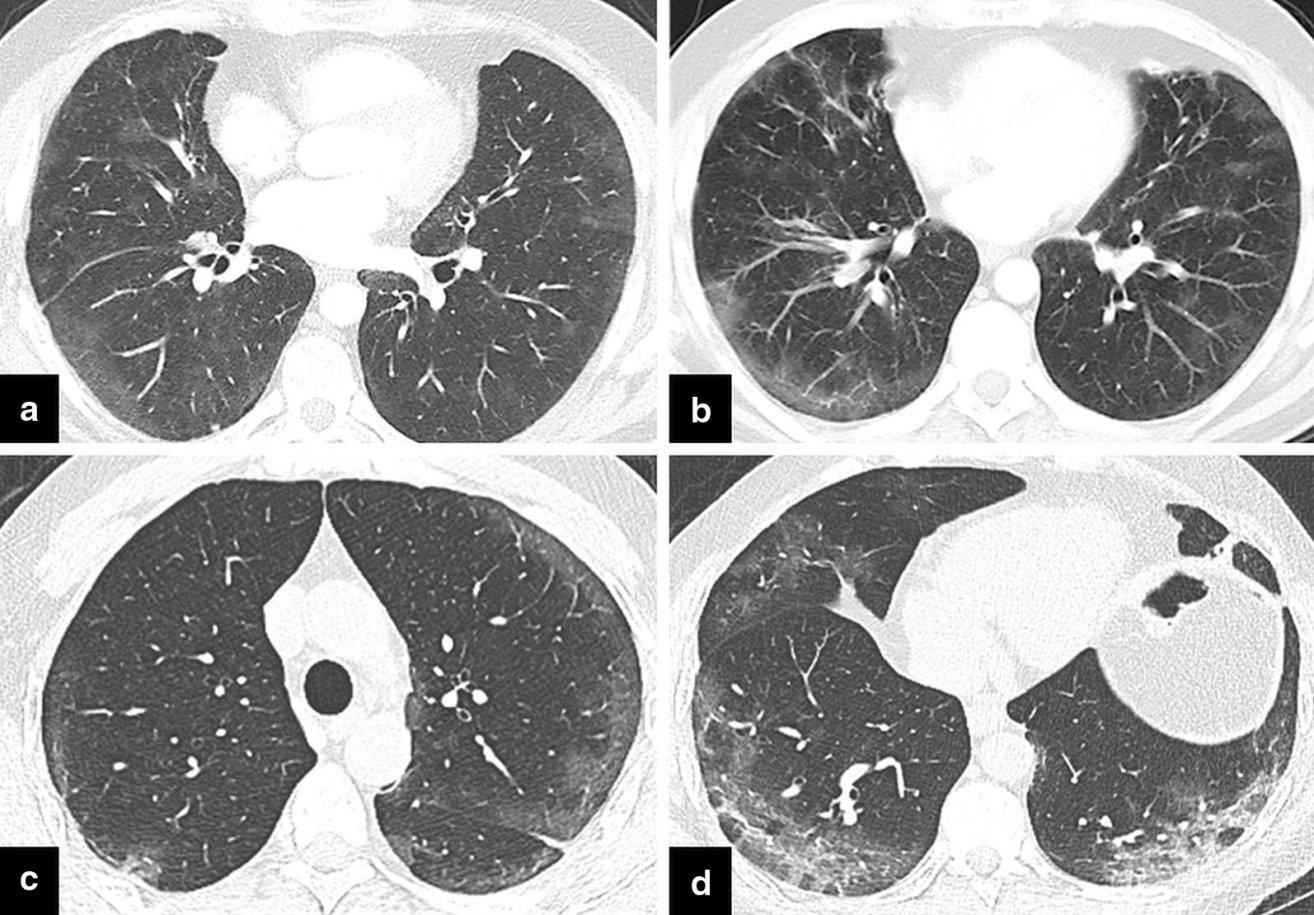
COVID-19 pneumonia-mimicking interstitial lung disease. a, b In desquamative interstitial pneumonia (DIP), bilateral peripheral GGOs are observed. c, d Axial CT image showing bilateral subpleural GGOs and opacities in a patient diagnosed with COVID-19
